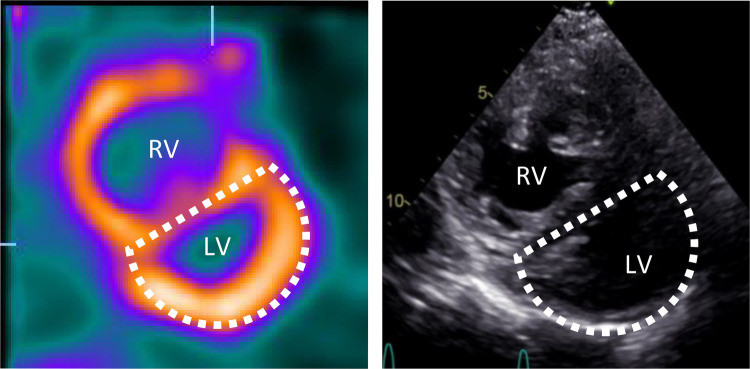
Movahed's sign on myocardial perfusion SPECT and D-shaped left ventricle on echocardiography. Short axis myocardial perfusion SPECT images at rest (left) and short axis view on transthoracic echocardiography (right) show the D-shaped left ventricle. SPECT, single photon emission computer tomography; RV, right ventricle; LV, left ventricle.
